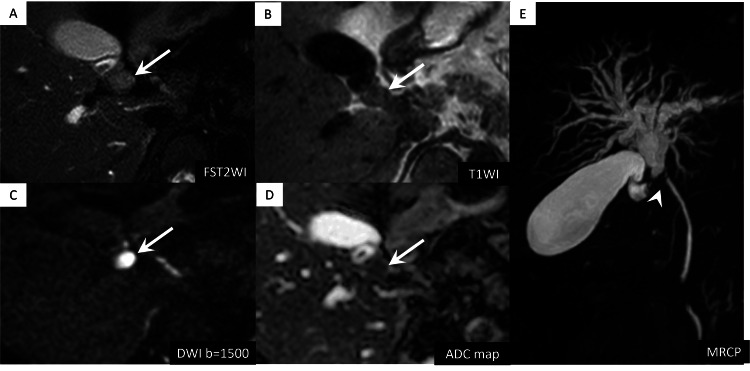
Abdominal magnetic resonance imaging (MRI) findings. (A) and (B) The nodule (arrows) shows mildly high signal intensity on T2-weighted imaging (T2WI) and low signal intensity on T1-weighted imaging (T1WI).
